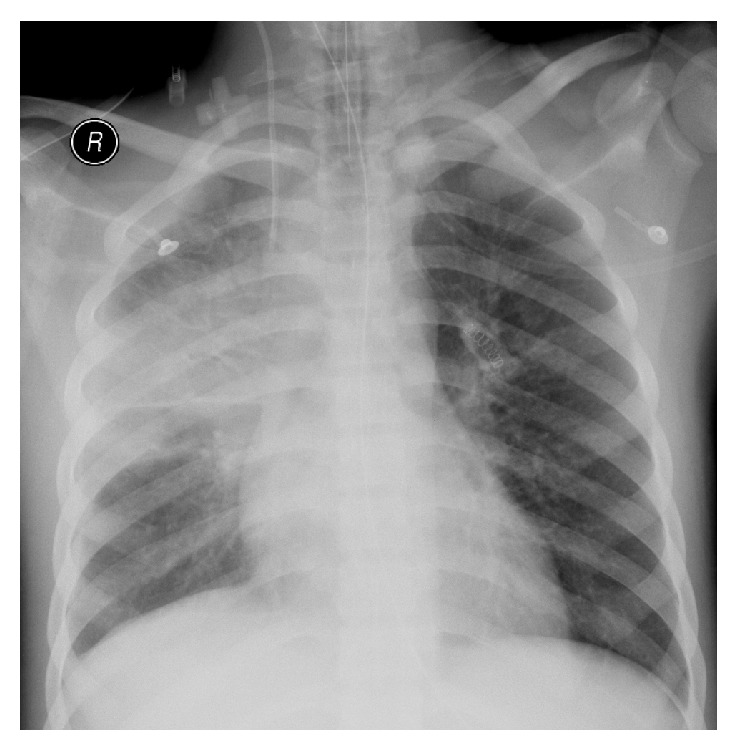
Chest radiograph showing a right mid zone consolidation and a slightly blunt right costophrenic angle.
